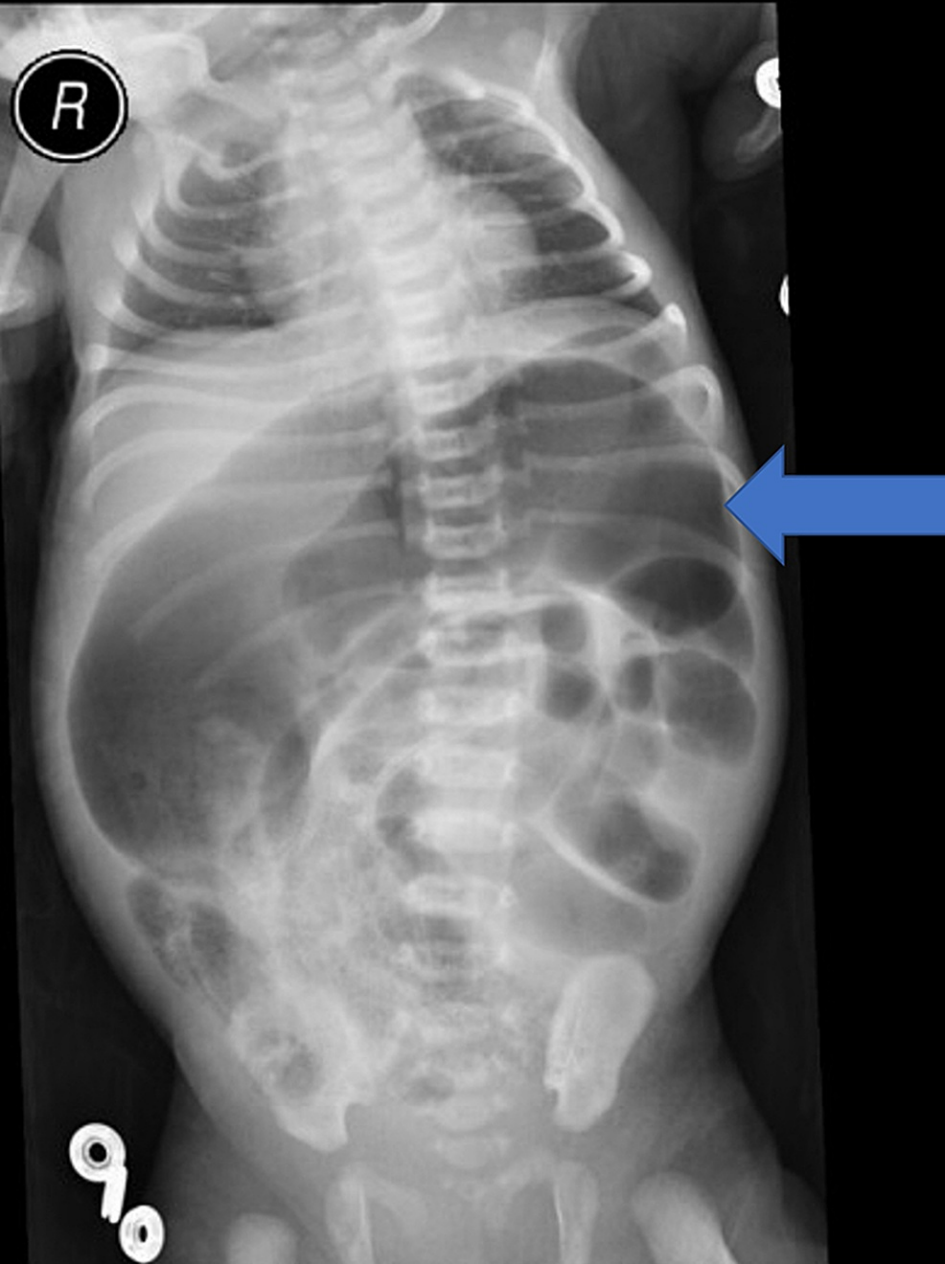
Plain abdominal x-ray showing hugely dilated bowel loops (more likely colon) and air-fluid levels with no gas distally at the rectum. Arrow: dilated left-sided bowel loops, R: right.
